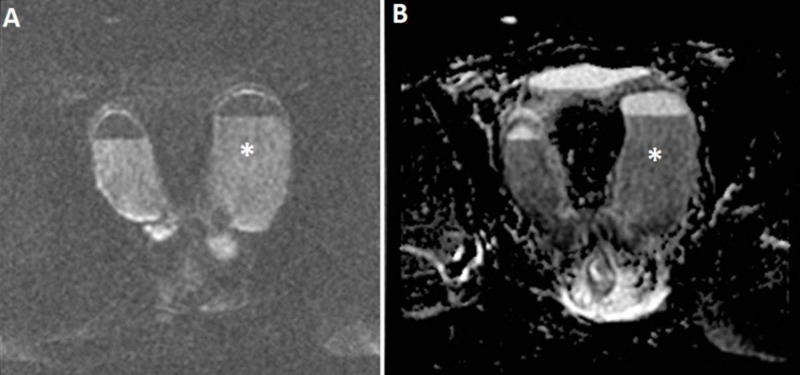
Coronal DW-MRI at the b-value of 800 s/mm2​​​​​​​ (A) and ADC map (B) of the cystic changes. The bottom level (asterisk) shows a restriction of diffusion. ADC, apparent diffusion coefficient; DW-MRI, diffusion-weighted magnetic resonance imaging
